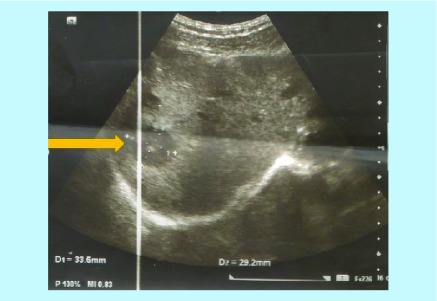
Ultrasonography showing the largest lesion in the liver 24 months postsurgery.
